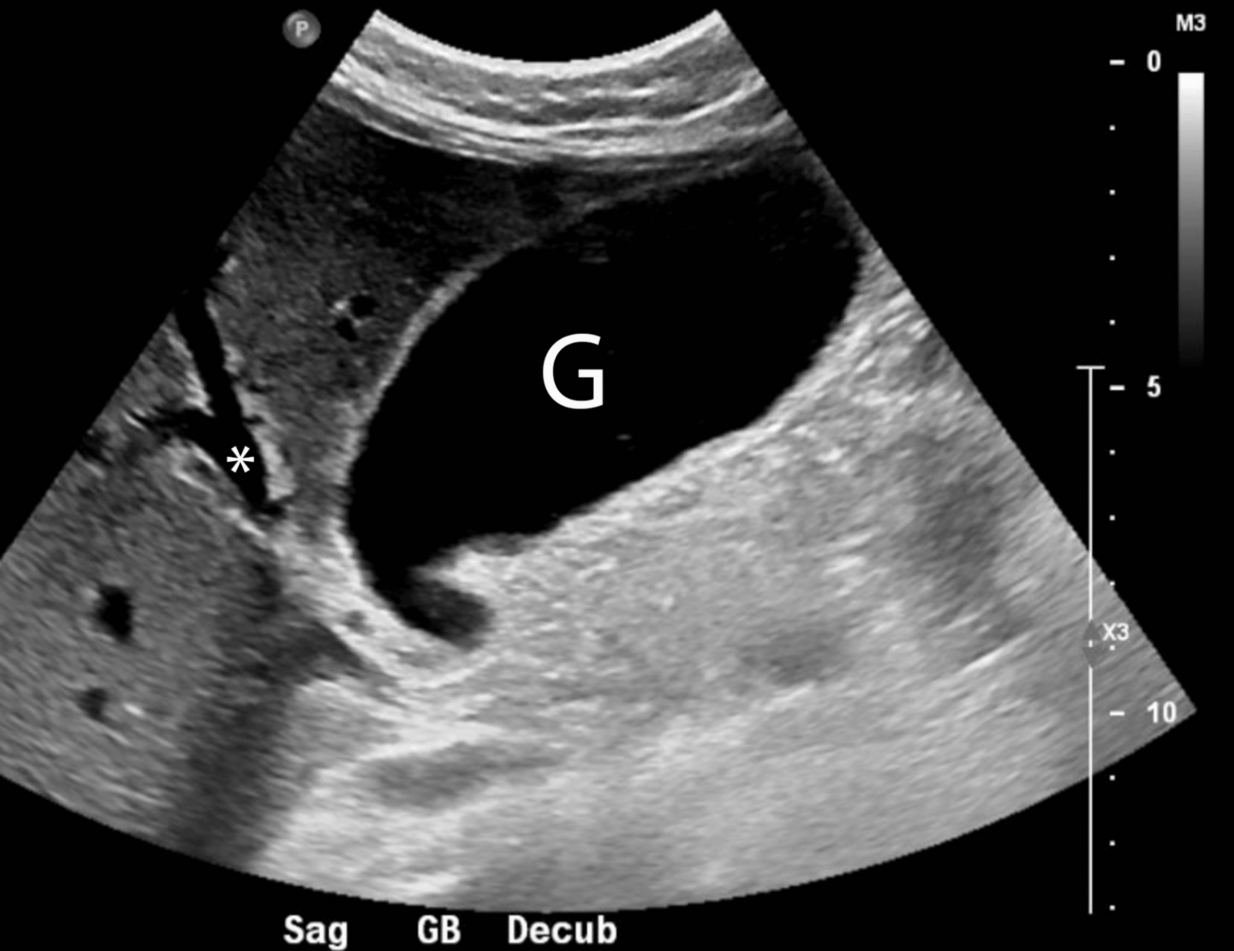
Right upper quadrant ultrasound The ultrasound demonstrates distention of the gallbladder (G) with no obvious obstruction. The common bile duct is measured to be 5.4mm in diameter (*).
